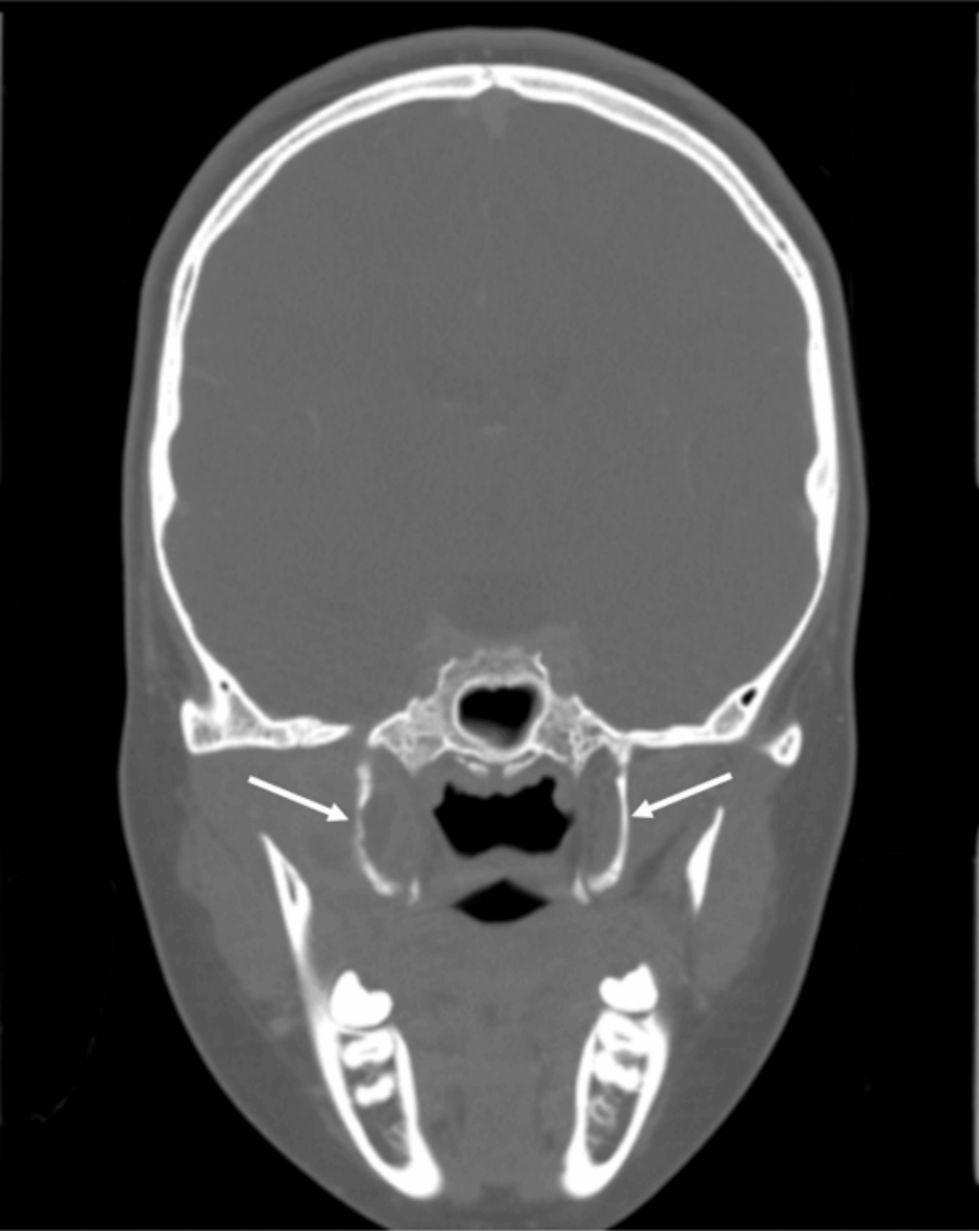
Third hospital admission. Coronal slice CT head with contrast showing early erosion of the lateral pterygoid plates, worse on the right
